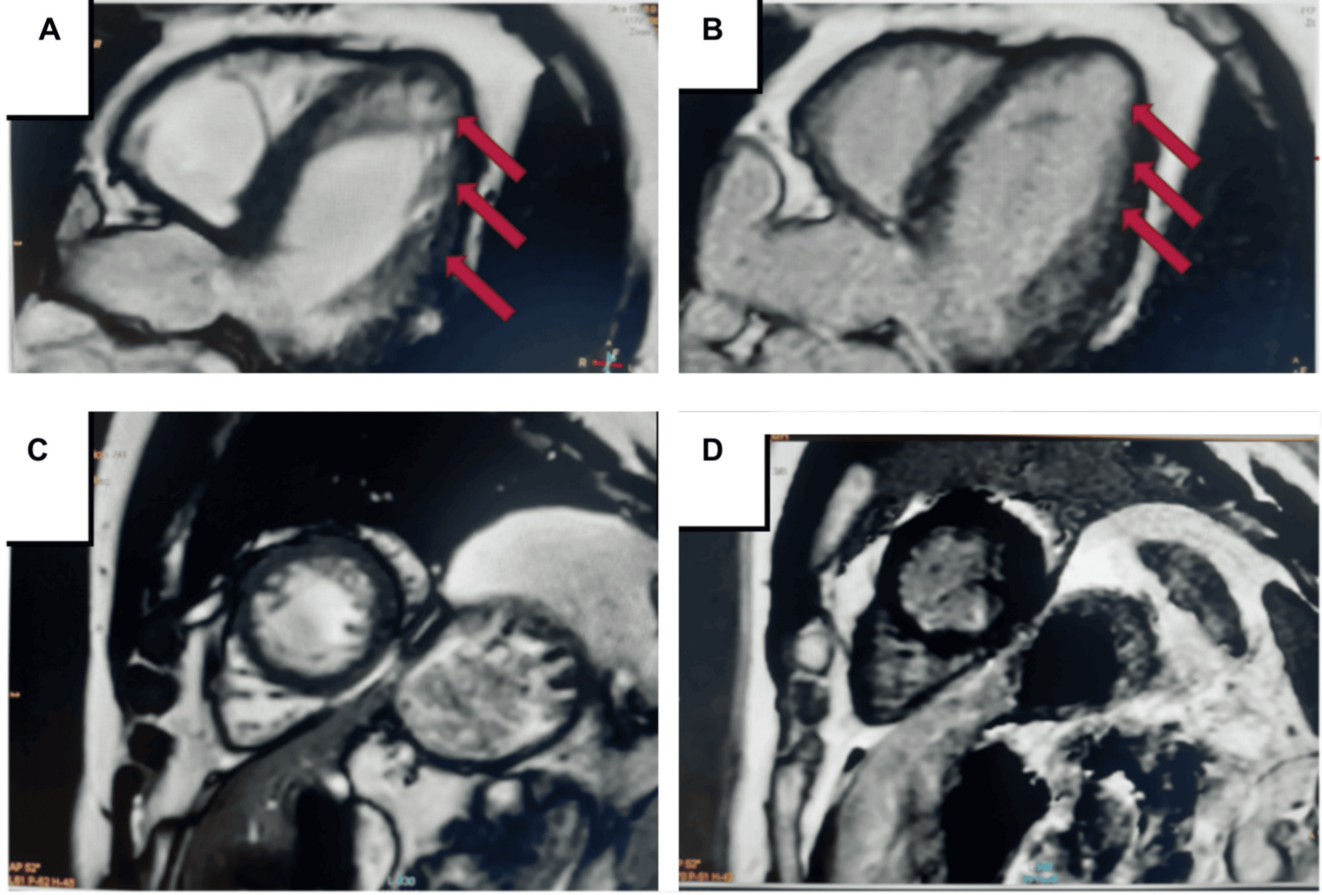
MRI images Ischemic heart disease with moderate systolic dysfunction of the left ventricle, left ventricular ejection fraction (LVEF) 50%. Red arrows indicate areas of late gadolinium enhancement; preserved myocardial viability is evident in the left anterior descending artery (LAD) territory. No endocardial thrombus was observed. No endocardial thrombus observed. A,B: 4-chamber view; C,D: Short-axis view.
